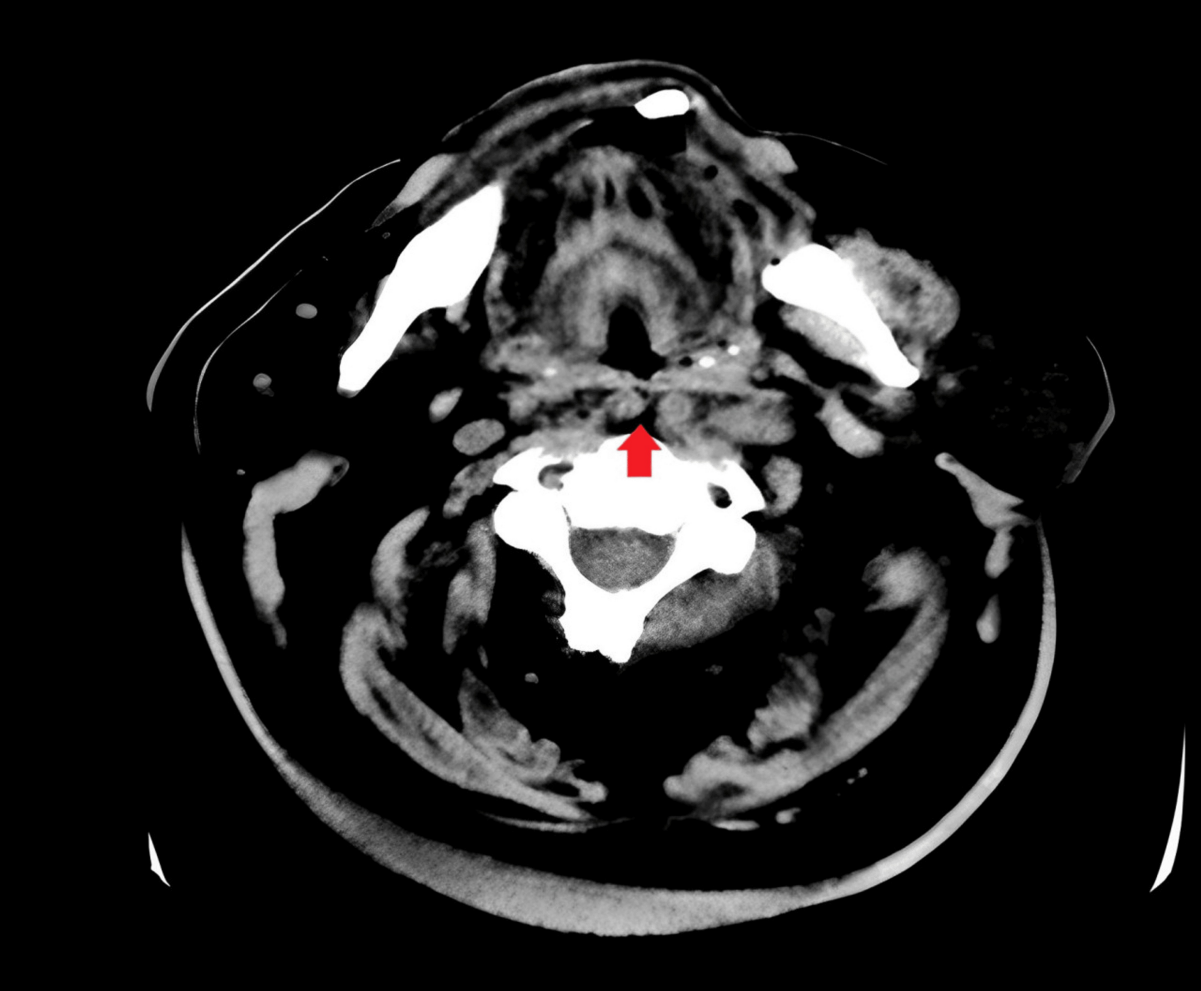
Computed tomography angiogram (CTA) showing bilateral aberrant course of the internal carotid arteries
